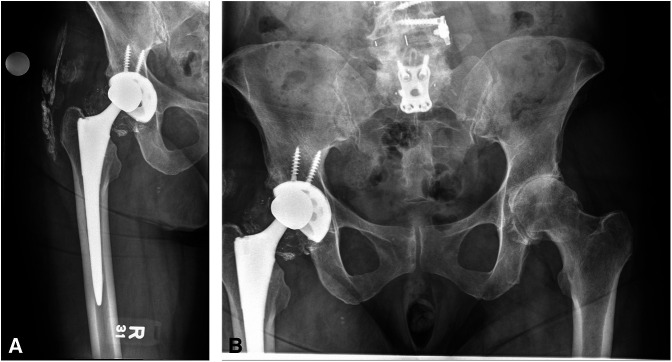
A, AP radiograph of the hip with an eccentric position of the femoral head and metallosis of the soft tissues. B, AP radiograph of the pelvis demonstrating the same changes.
